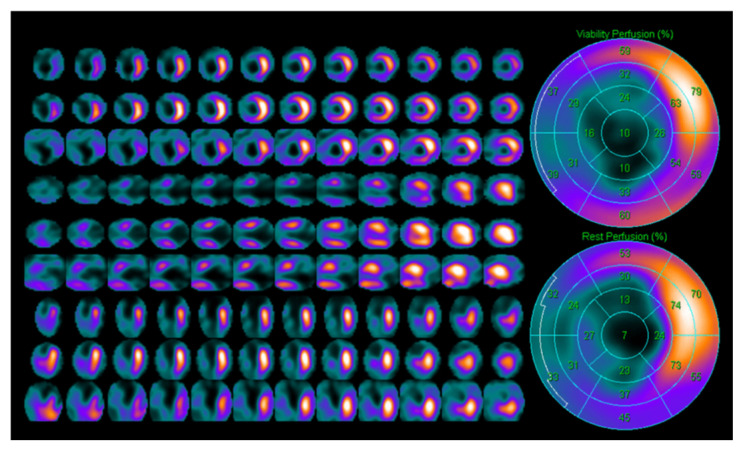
Rest–redistribution Thallium-201 SPECT imaging showing delayed uptake in the inferior wall, indicative of viable myocardium post-infarction. Adapted from Santoyo-Saavedra et al., 2022, under open-access terms [29].
